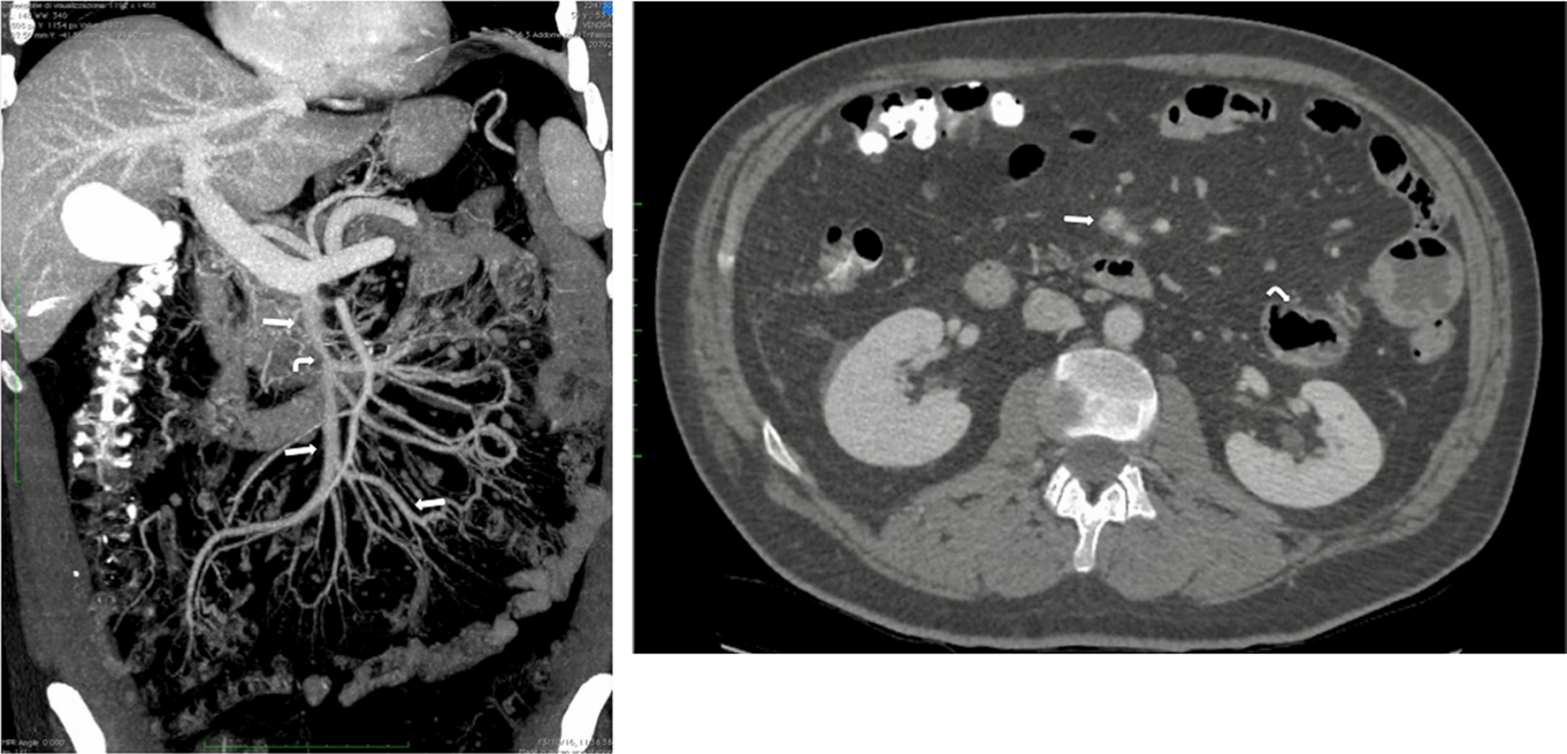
a Pre-discharge postoperative CT confirms recanalization of both main trunk and side branches of the SMV (arrow); a residual thrombosis is still evident into the SMV lumen (curved arrow). b Pre-discharge postoperative CT shows resolution of jejunal thickening (curved arrows) and recanalization of the SMV main trunk (arrow)
